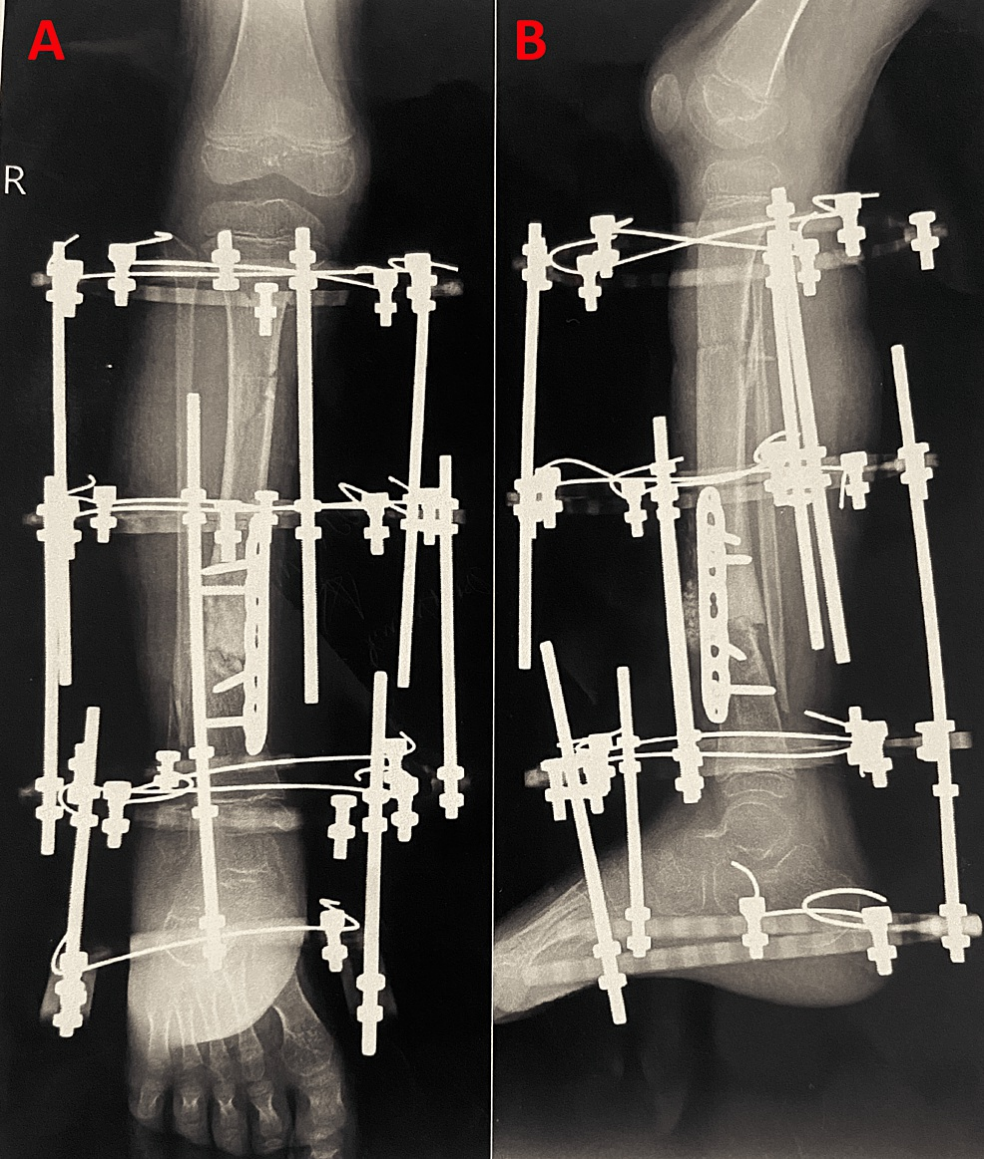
Radiograph showing Ilizarov fixation. Anterior-posterior and lateral radiographs that were taken postoperatively of a patient with fixators in Ilizarov fixation.
